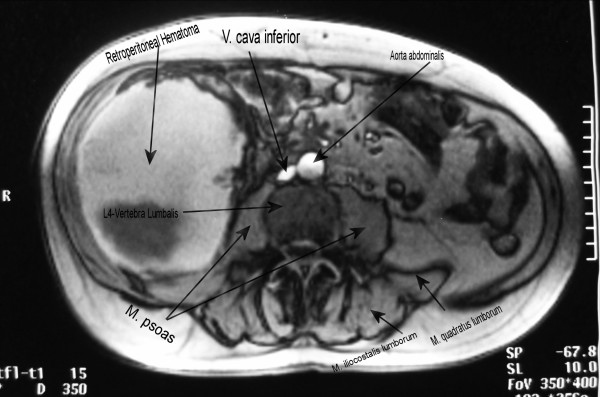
MRI – transverse plan (L4) with IV contrast gadolinium-BOPTA, revealing a well-defined mass, a huge retroperitoneal hematoma.
